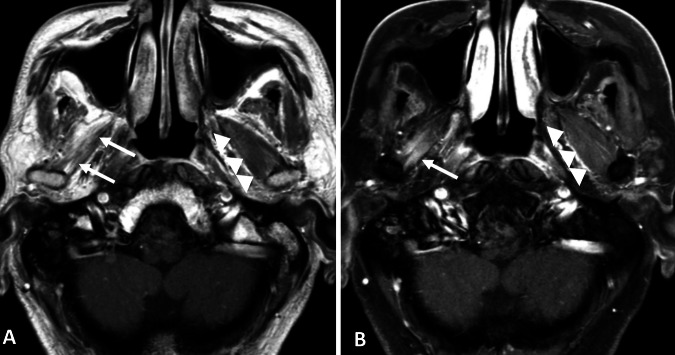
Post-treatment axial contrast-enhanced T1 (A) and fat-suppressed contrast-enhanced T1 (B) MRI images of a patient with nasopharyngeal carcinoma (NPC) treated with RT 4 years ago. The right lateral pterygoid muscle (arrow) displays diffuse enhancement signal and decreased size compared to the contralateral side (arrow heads), suggesting atrophy
